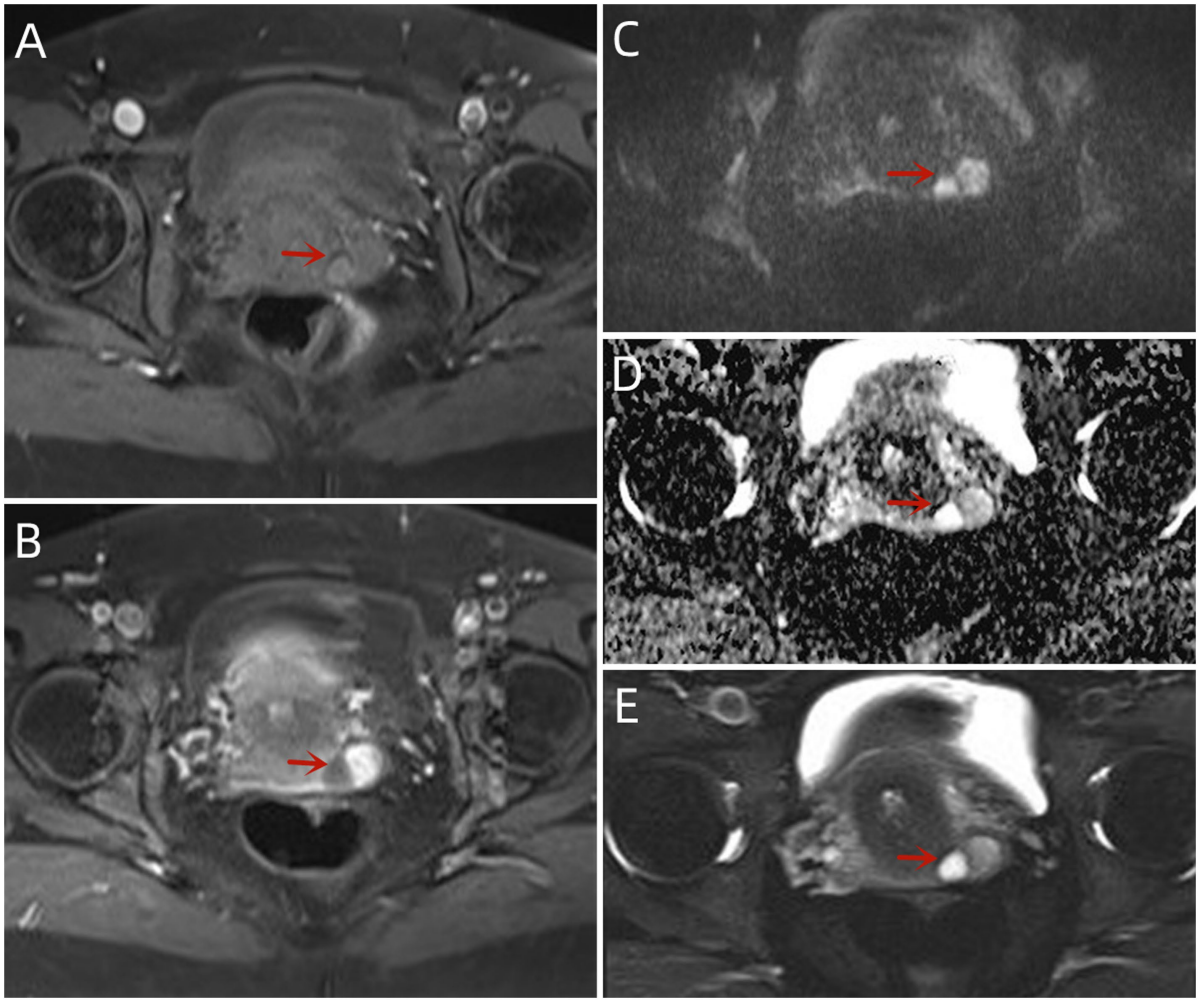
MRI findings of this case: (A) The lesions with the T2-FS sequence exhibited an uneven hypersignal. The right side exhibited a T2 hypersignal, similar to fluid. Magnetic resonance imaging findings of this case: (B,C) Both diffusion-weighted imaging (B800) and apparent diffusion coefficient lesions exhibited high signals with an unrestricted diffusion. (D) The T1-FS lesions had uneven and slightly hyperactive signals. (E) Enhancement of the left side of the lesion was observed after intravenous injection of contrast medium.
